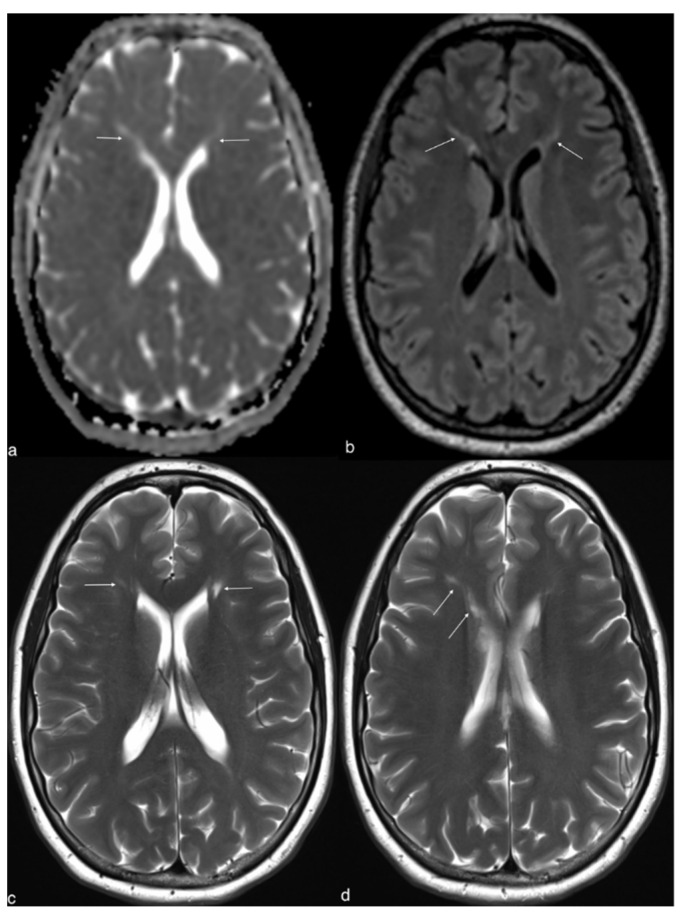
Brain MRI shows an increased ADC (arrows in (a)) associated with linear high signals lesions of the white matter in front of the frontal horns of lateral ventricles on FLAIR and T2 (arrows in (b–d)). ADC, apparent diffusion coefficient; MRI, magnetic resonance imaging.
